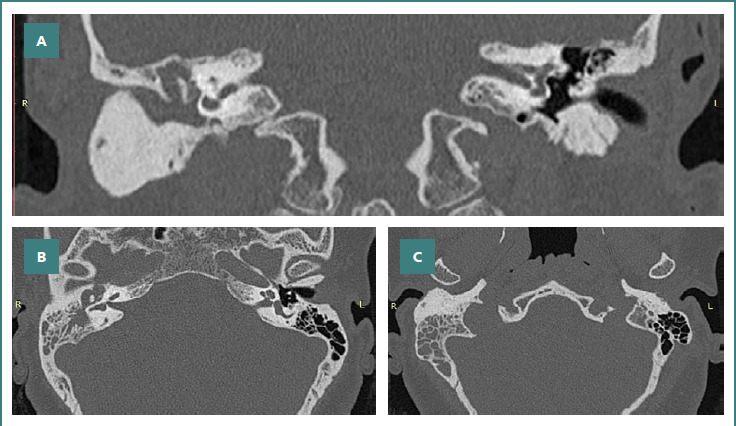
High-resolution computed tomography findings of the right temporal bone. A, Axial section showing a sessile osteocondensing mass arising from the anterior mastoid cortex, with complete opacification of the external auditory canal lumen and tympanic cavity. B, Coronal lateral section showing extension of the osteoma toward the mastoid apex, with scutum erosion and dense antral opacification. C, Coronal medial section showing preserved medial external auditory canal caliber, confirming that the stenosis originates from the lateral cortical osteoma.
